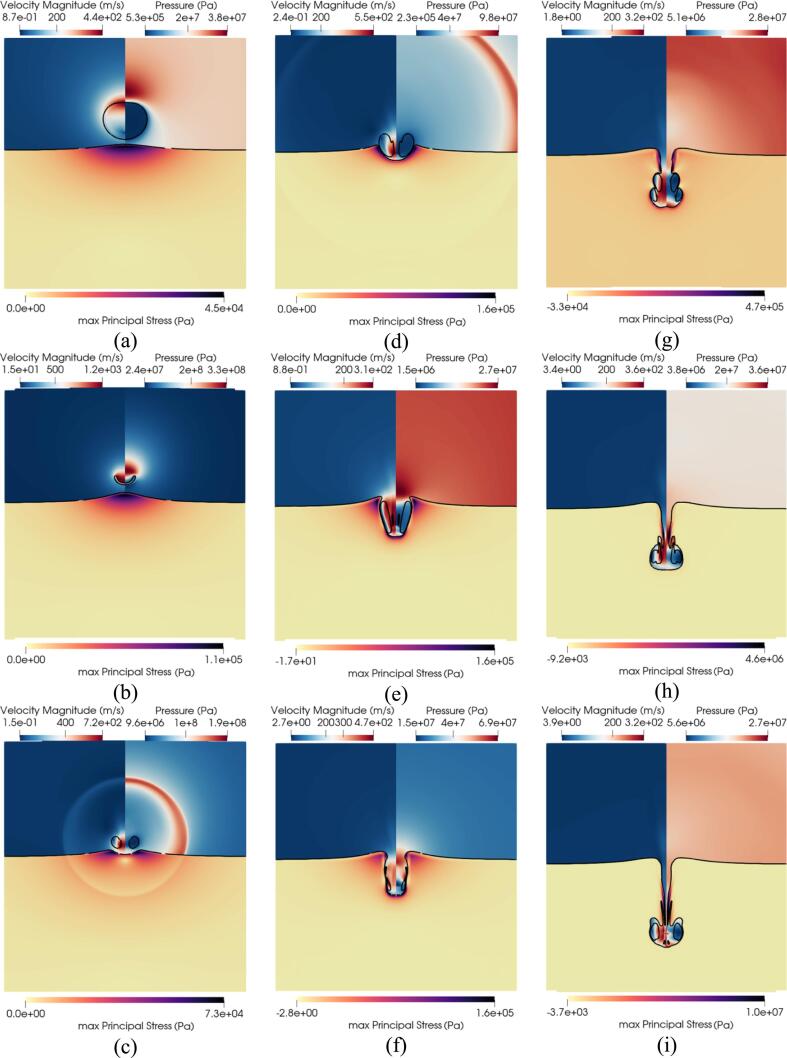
Ultrasound-induced collapse of an air bubble of $R_{0}=10\mathrm{\mu m}$ with an initial standoff $S_{d}\;=\;1.1\;R_{0}$ near gallbladder at different timesteps. The black isosurface separates the three materials and is defined by $\alpha_{k}=0.5.$ Upper left hand: contour of velocity magnitude. Upper right hand: contour of pressure. Bottom half: contour of the maximum principal stress.
